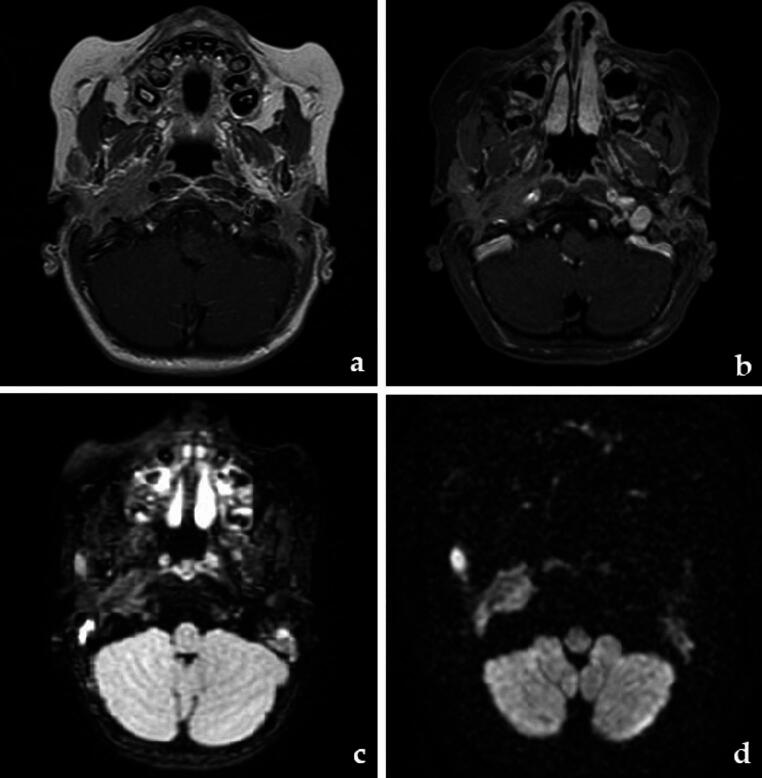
MRI scans of the iEMR. a) T1, b) T1 with contrast enhancement, c) FLAIR, d) DWI
